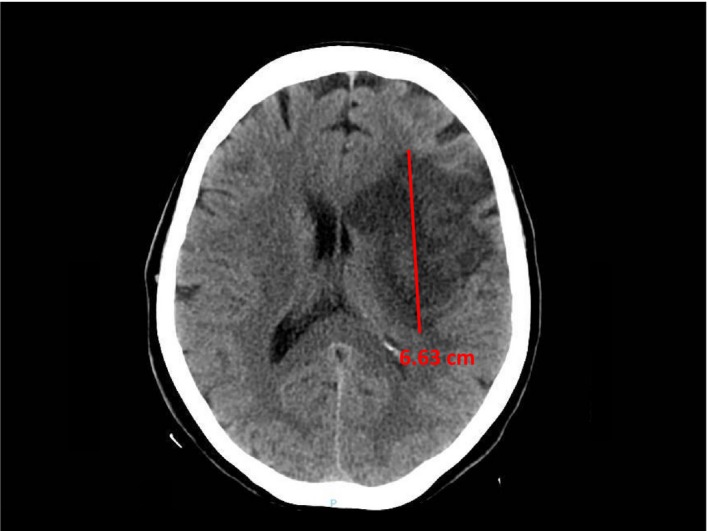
CT image showing an example of maximum cerebral lesion diameter measurement and mass effect
